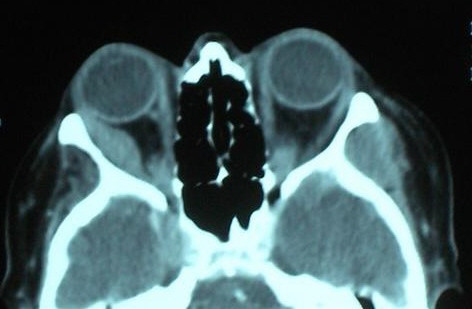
Axial computed tomography scan of the patient showing a mass in the right lateral rectus.
